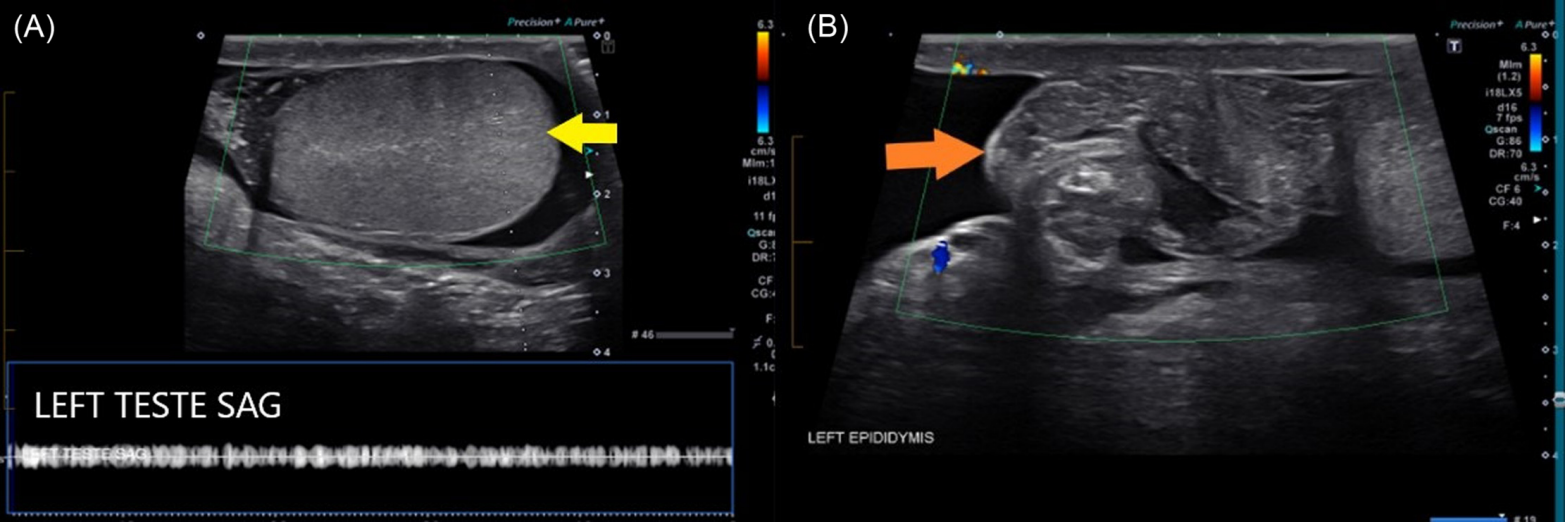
Arterial and venous flow in the left testicle and epididymis under Doppler ultrasound. Note the lack of flow in both the left testicle (yellow arrow) (A) and left epididymis (orange arrow) (B). There was a heterogenous mass (orange arrow) superior to the left testis without blood flow, consistent with tangled vasculature (B). A discussion with the radiologist determined that the spectral waveform was artifact and not venous flow (A). *TRANS*, transverse view; *SAG*, sagittal view.
